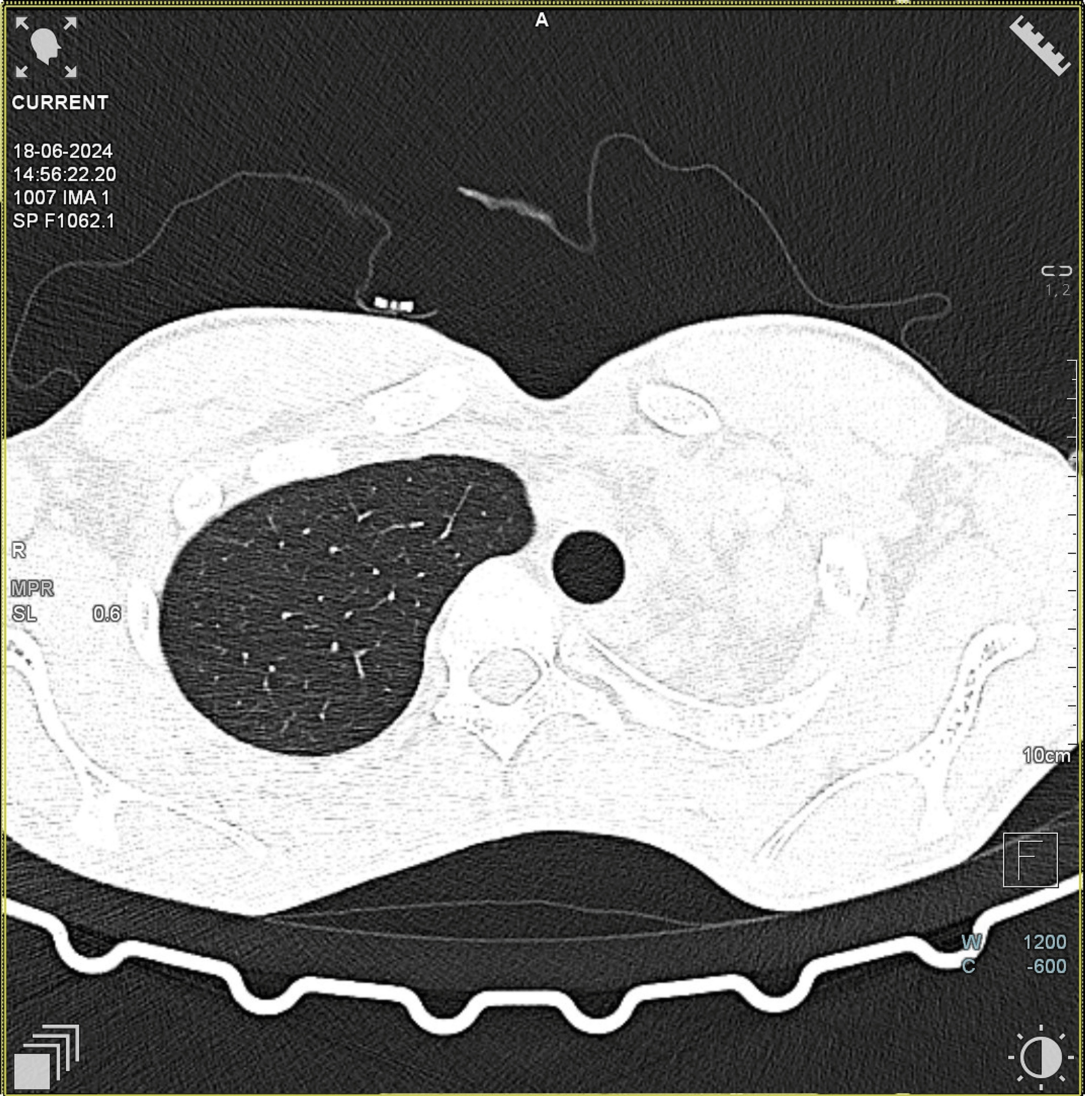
CT chest-axial section at T3 level The image shows the absence of the left upper lobe CT: computed tomography
